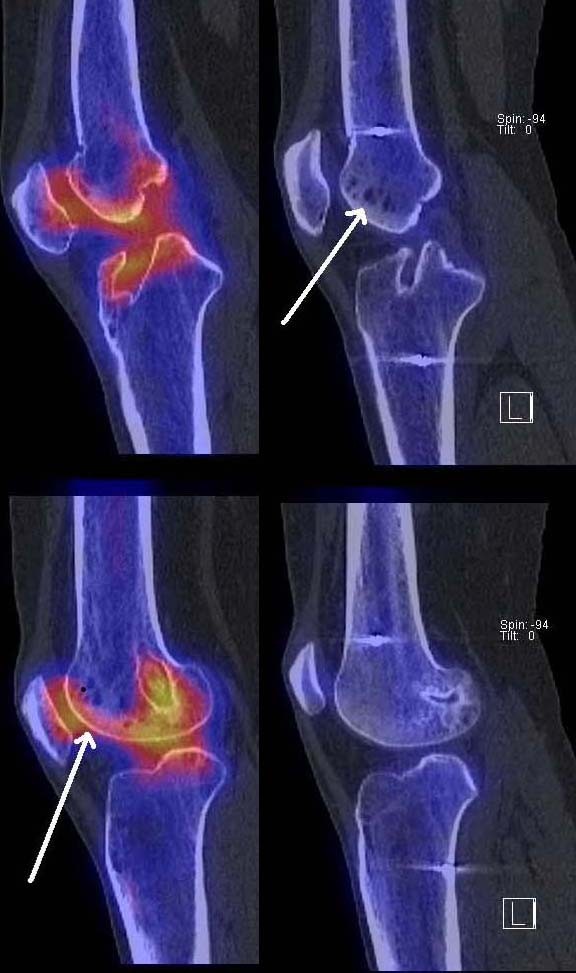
Sagittal, axial and coronal reformation of right and left knee in 99mTc-HDP-SPECT/CT showing increased tracer uptake within the femoral and tibial tunnel and patellofemoral joint of the left knee. The right knee did not demonstrate any areas of increased tracer uptake.
